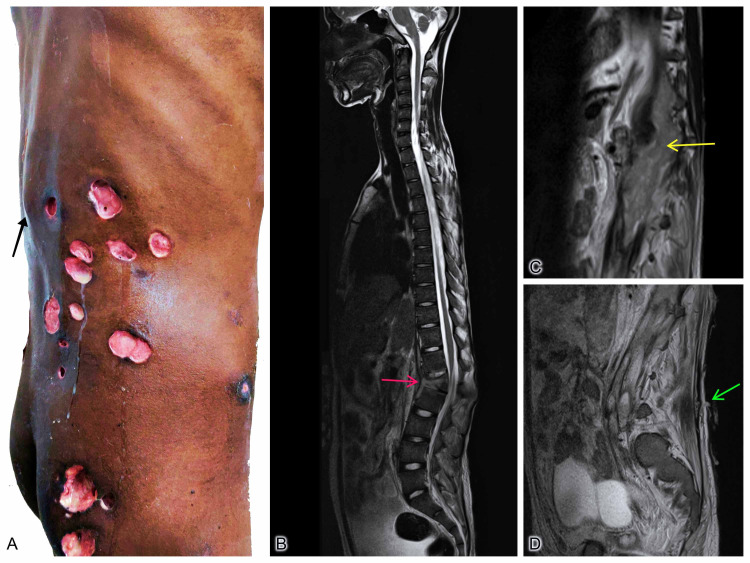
(A) “Swiss Cheese Loin” appearance with multiple ulcers over the right flank and buttock with active pus discharge; (B, C, D) T2 MRI sequences showing the sites of the lesion. Black arrow (A) points to the gibbus deformity at L1 level. Red arrow (B) shows kyphotic angulation due to anterior wedge collapse of L1 vertebral body with normal posterior elements. There is kinking of dura which is abutting the cauda equina nerve roots at this level. Yellow arrow (C) shows abscess collection in the paraspinal region, which is extending into the bilateral psoas regions. Green arrow (D) shows a real-time image of active pus discharge from the cutaneous sinus. MRI, magnetic resonance imaging
